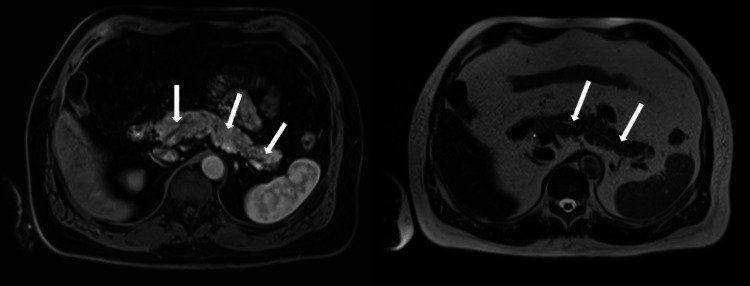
T1-weighted magnetic resonance imaging Pancreatic body and tail without parenchymal changes suggestive of chronic pancreatitis (at left); pancreatic body and tail, and duct without dilatation (at right).
